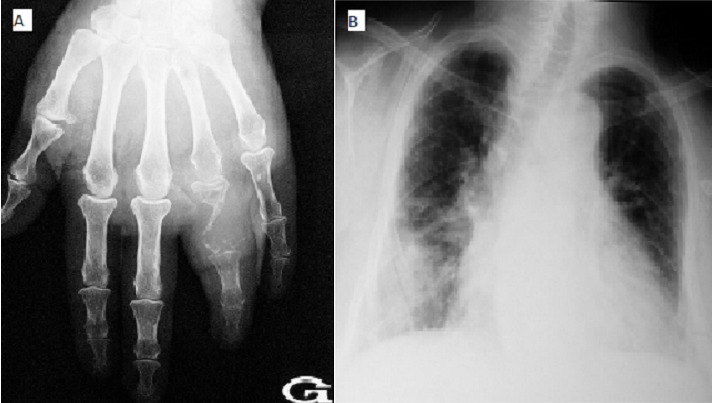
(A) radiographie de la main montrant une ostéolyse de la 1^ère^ phalange du 4^ème^ doigt avec rupture corticale sans réaction périostée; (B) radiographie du thorax révélant des adénopathies hilaires bilatérales avec un infiltrat interstitiel
